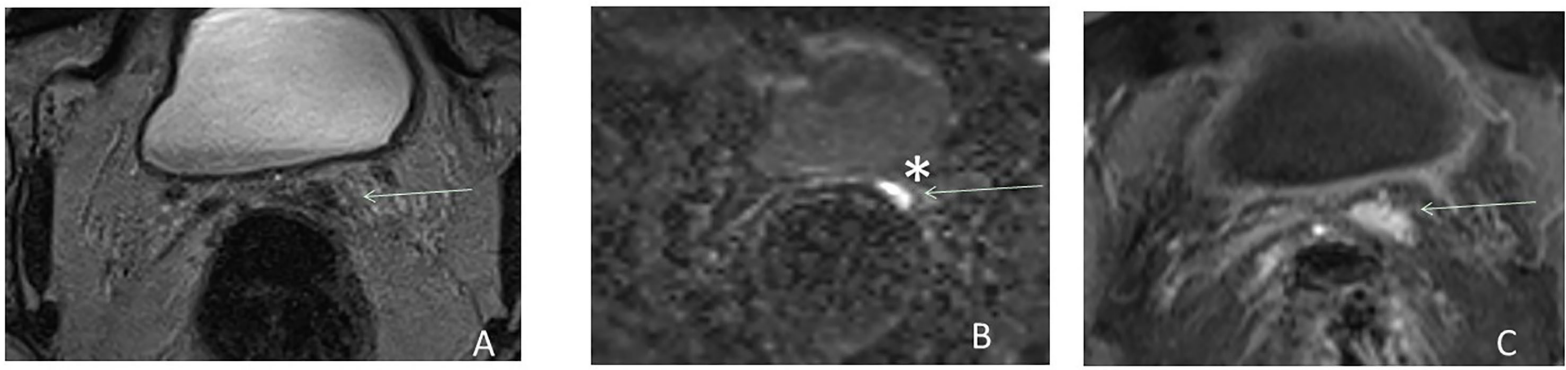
Postsurgical recurrence after RP. Axial T2W MRI (A), b2000 DW MRI (B), and DCE MRI (C) show the benefit of functional imaging to detect local recurrence in the left seminal vesicle bed (arrow) and particularly the benefit of high *b*-value ^*^. *Highlight the benefit of high b value.
